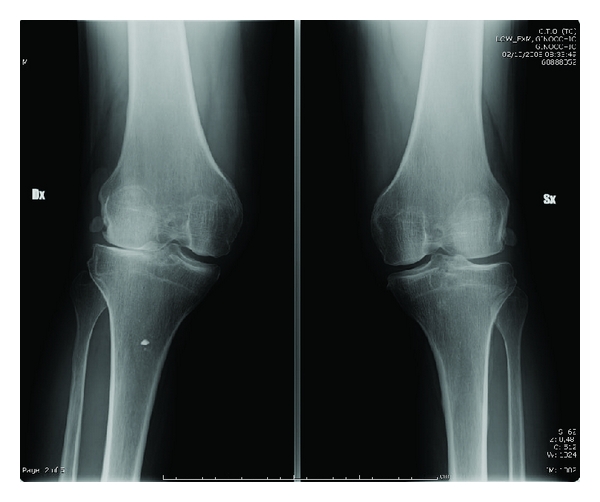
Roentgenograms of bilateral knees in AP, showing laterally displaced patellae with initial degenerative tibiofemoral arthritis.
